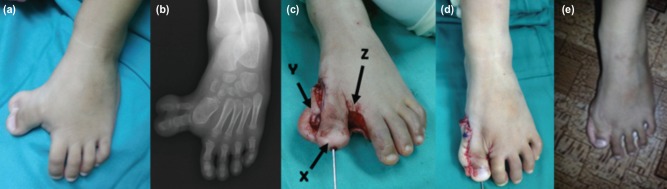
(a,b) Pre-operative photograph and radiograph of case No. 2 show polysyndactyly and congenital hallux varus of the left big toe. (c) The realigned big toe ‘x’ held with K-wire after medial soft tissue release with plantar based skin flap ‘y’ after filleting of bone in the extra digit and resection of the skin in the first web space ’z’. (d) The extra skin sutured to cover the medial skin defect and the first web space closure. (e) Post-operative photograph after 3 years.
